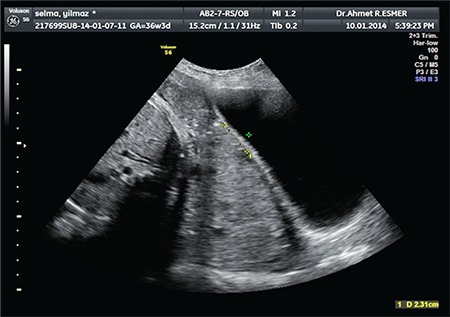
Transabdominal ultrasonographic view of total placenta previa
